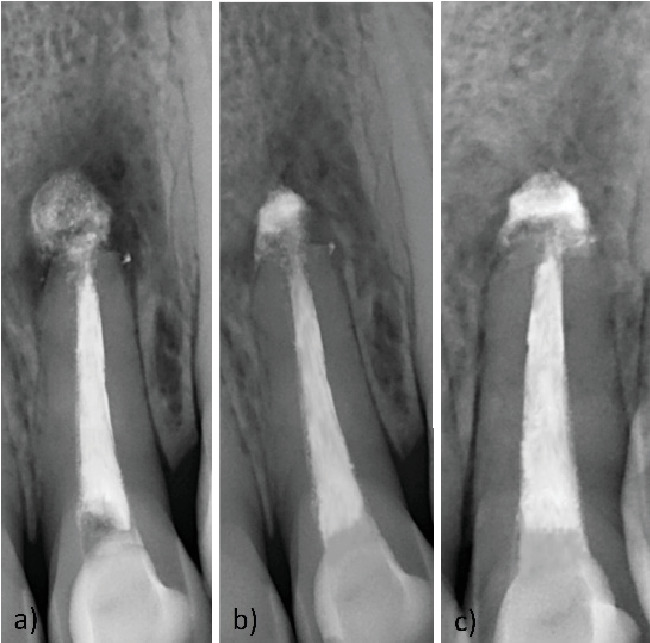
Clinical features of Case Report 1. (a) Postoperative intraoral radiograph, a notable portion of extruded MTA. (b) Six-month follow-up, note gradual healing of the periapical lesion. (c) Twenty-month follow-up, note the periapical osseous healing with gradual resorption and reorganization of extruded MTA.
